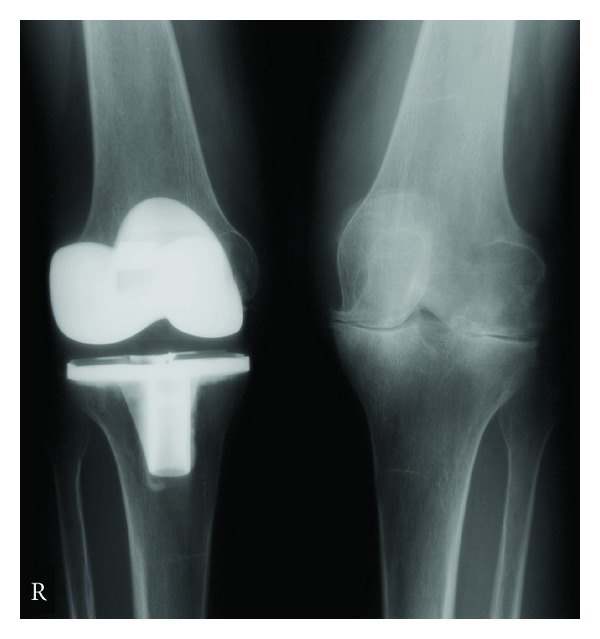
The same patient from Figures 1–3, now 22 months after right TKA was performed. The PA radiographs are seen with improved joint alignment in the right knee. The patient is doing well, walking without pain in the right knee, and is now being considered for a left TKA.
